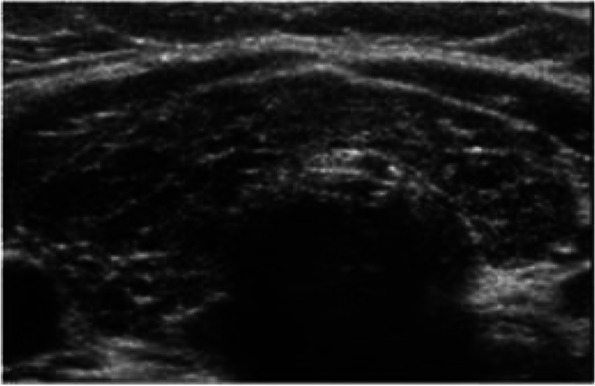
Gray-scale ultrasound images show heterogeneous thyroid parenchyma in a Group II participant
